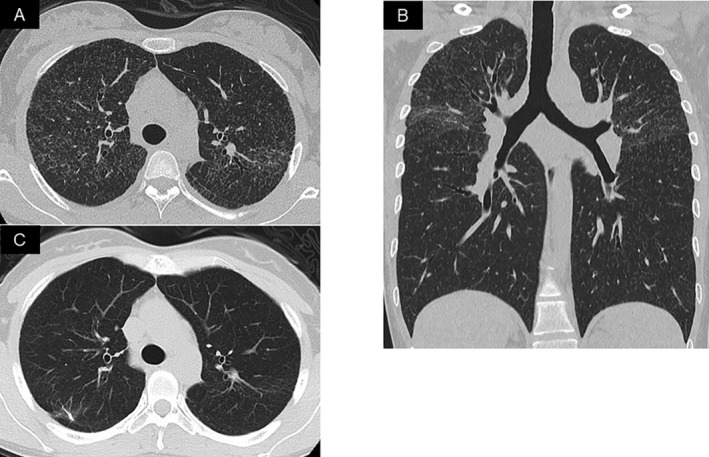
Chest high‐resolution computed tomography (HRCT) revealed miliary opacities in bilateral upper lobes predominance. (A) Transverse section on chest HRCT and (B) coronal images of chest CT. (C) At three months, after corticosteroid therapy, miliary opacities in both lung fields markedly improved.
